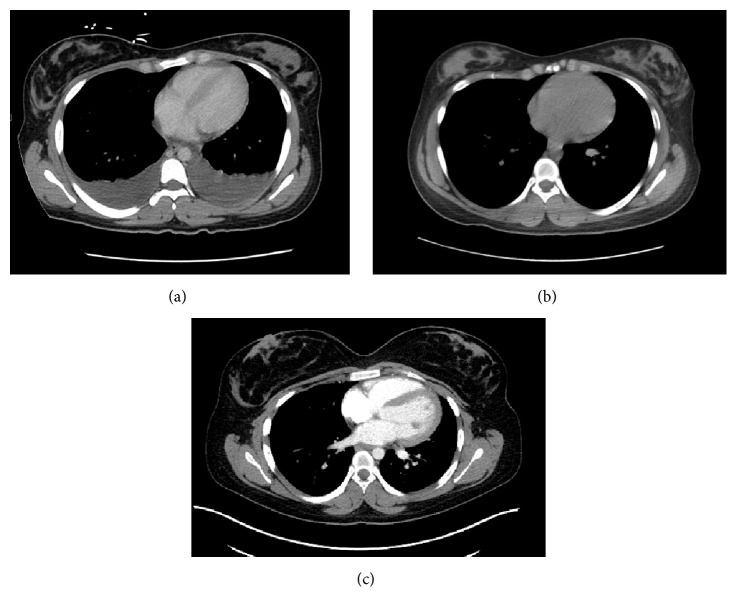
Contrast enhanced CT, axial view, upper chest. (a) Completed October 2016 with benign findings. (b) Completed November 2016 with moderate size bilateral pleural effusions. (c) Completed June 2017 with benign findings.
